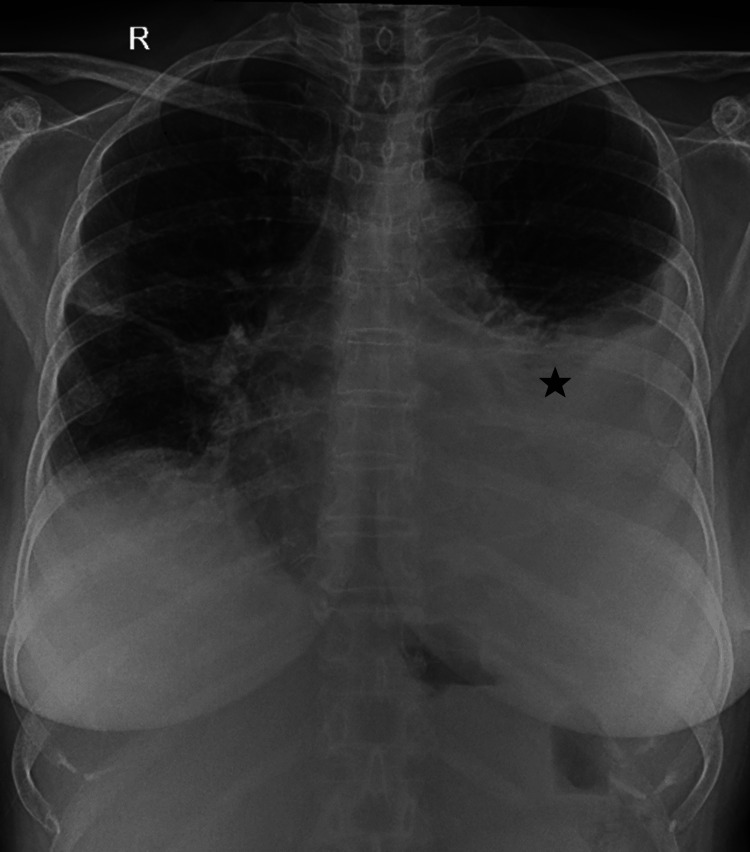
Chest x-ray revealing new-onset left pleural effusion (black star).
